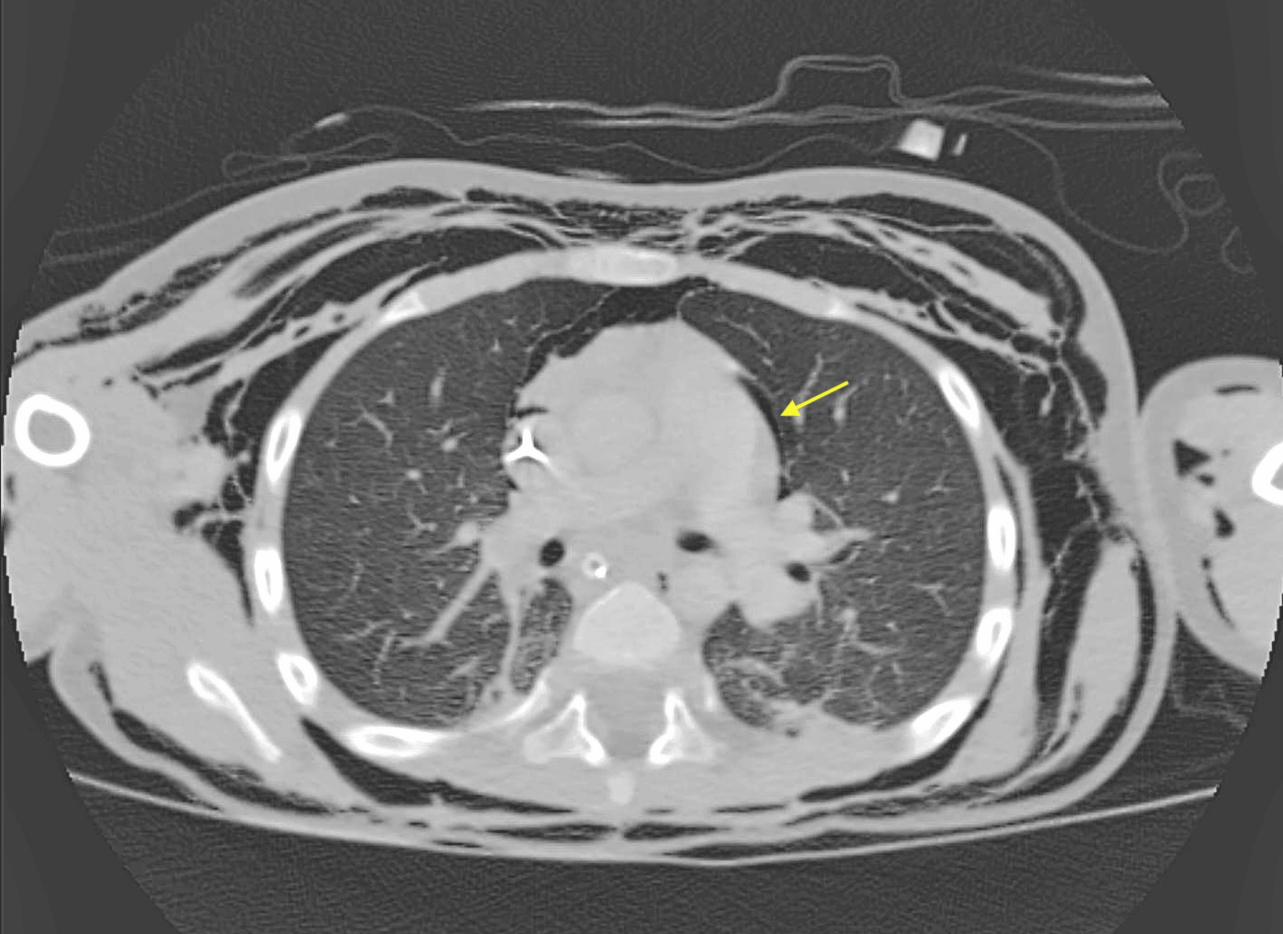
Axial CT Chest demonstrating left-sided pneumothorax (yellow arrow) This image highlights the presence of a small but clinically significant pneumothorax seen after tracheocutaneous fistula closure.
